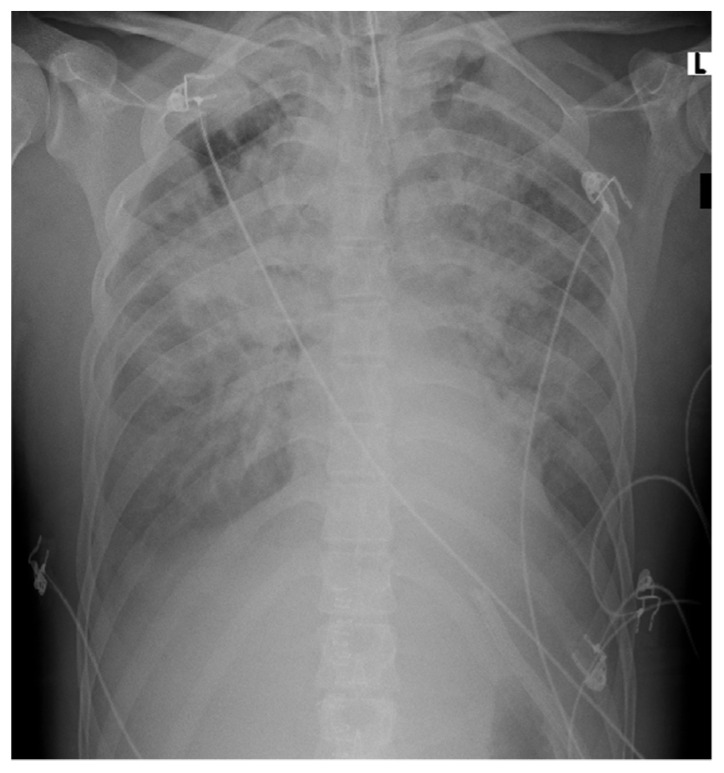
Patient chest X-ray. (L) left side of the patient.
